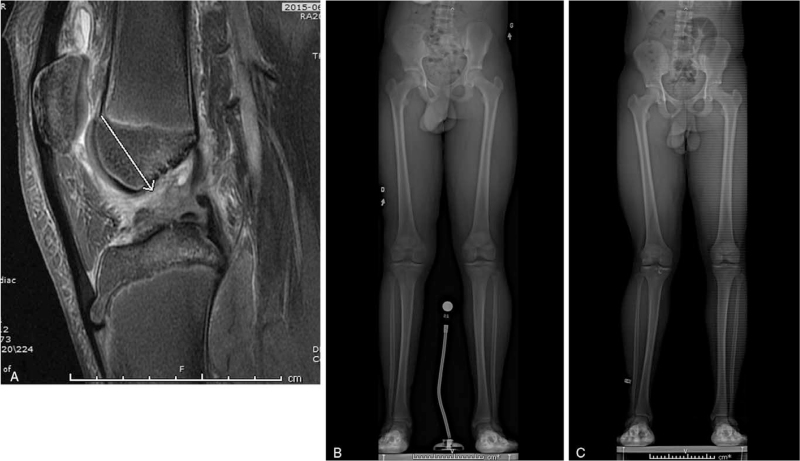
Preoperative MR imaging (a) and EOS radiograph (b) showing a 5 mm limb length discrepancy. At 3 years postoperative the EOS radiograph of the operated limb (c) shows 6 degrees of valgus.
